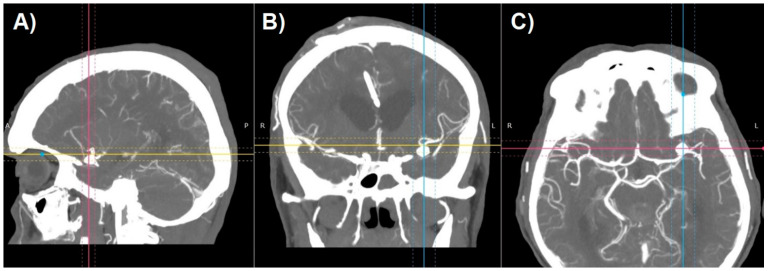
Maximum Intensity Projection reconstruction of CT-angiography of patient with cerebral aneurysm in (A) sagittal, (B) coronal, and (C) axial plane.
